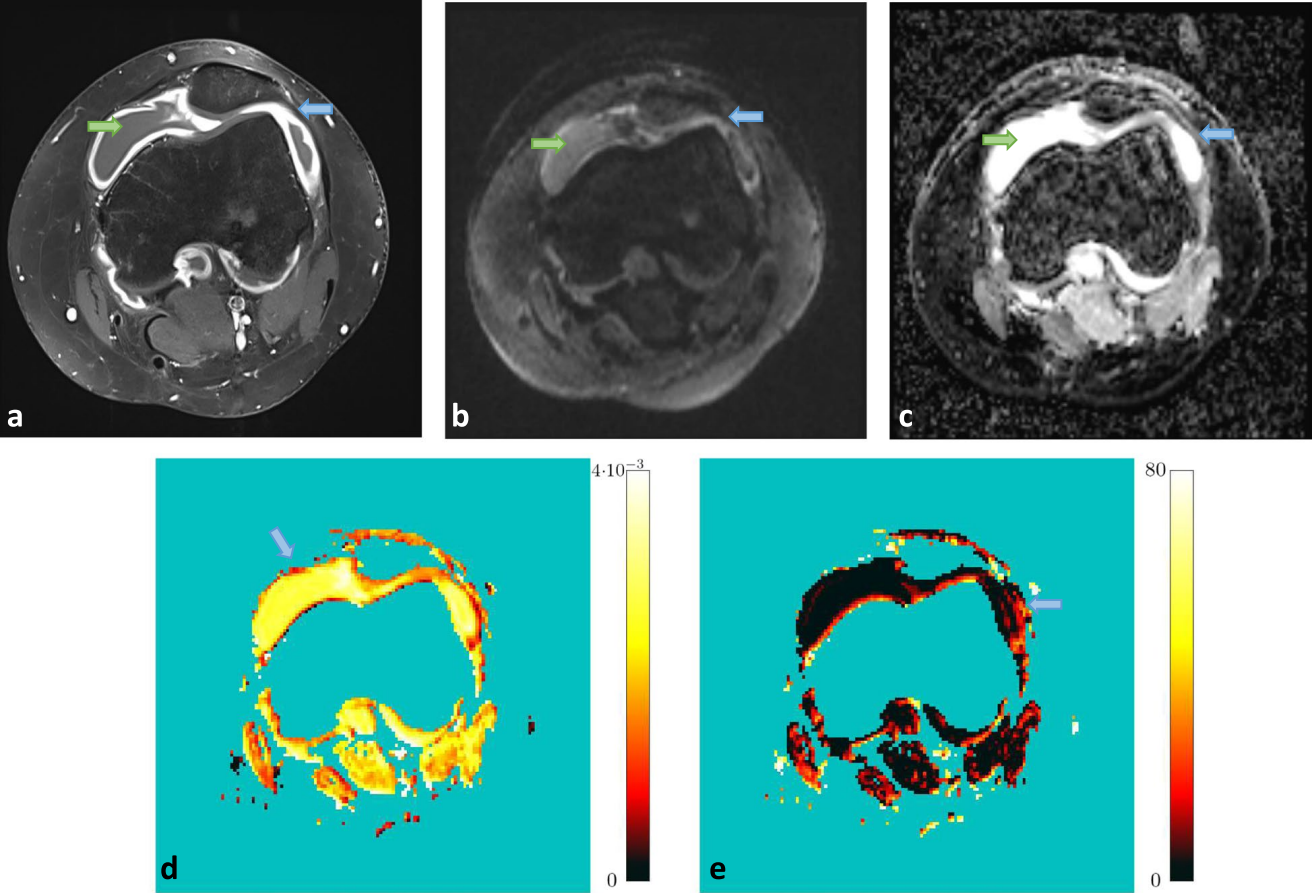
Persisting synovitis of the left knee joint under anti-inflammatory treatment in a 20 year-old male patient (patient 2 in Table 2) with known juvenile idiopathic arthritis (JIA). a) Contrast-enhanced T1-weighted MRI section showes diffuse synovial thickening and contrast-enhancement (blue arrow) along with marked joint effusion (green arrow), b) corresponding DWI at b = 1000 s/mm^2^ delineates high signal synovial layer (blue arrow) from low signal effusion (green arrow). c) ADC-map, d) masked D-map with colour-encoded diffusion ranging 0 - 4 × 10¯^3^ mm/s^2^ and e) f-map with colour-encoded perfusion fraction ranging 0 - 80% demonstrate restricted diffusivity and increased perfusion fraction of the synovial layer (blue arrows)
